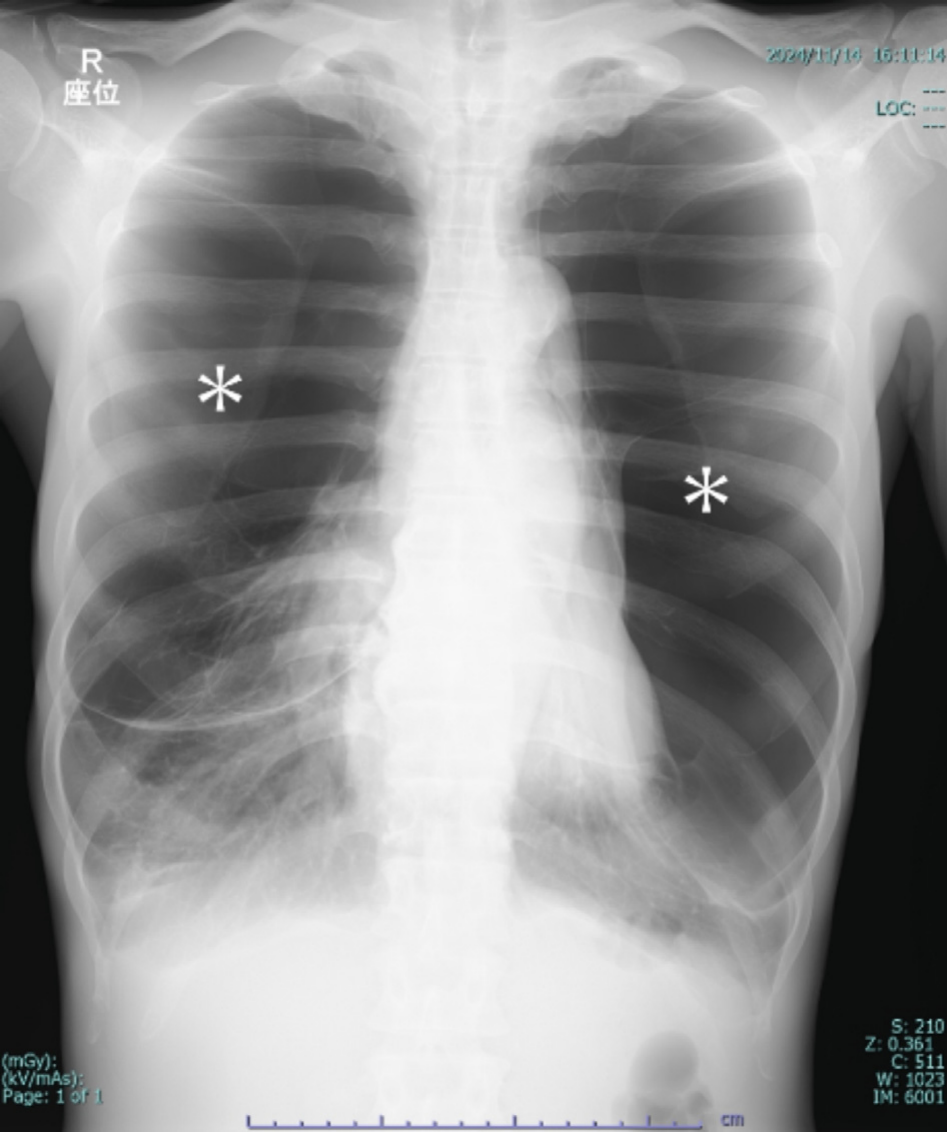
Chest radiography showing increased lung translucency and absent vascular markings in the upper and middle lung fields bilaterally (asterisks).
